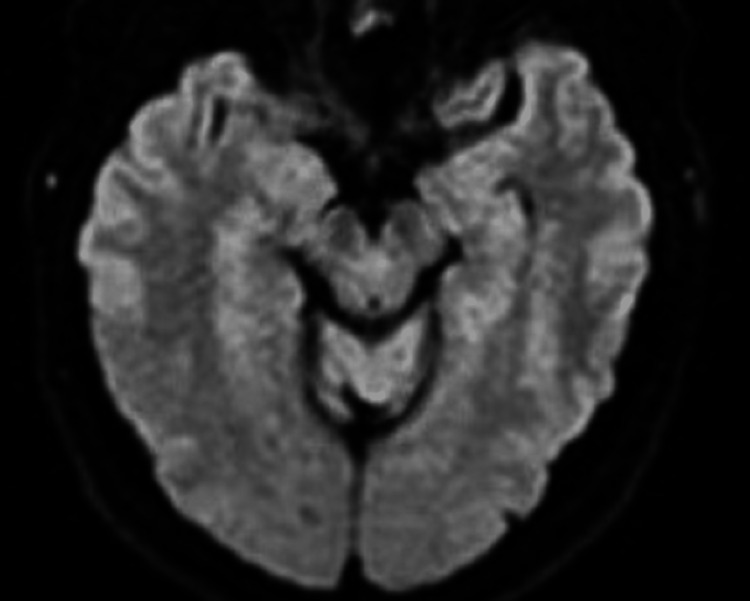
Magnetic resonance diffusion-weighted imaging (DWI) showing the same area, without any apparent residual findings, eight months after the episode
